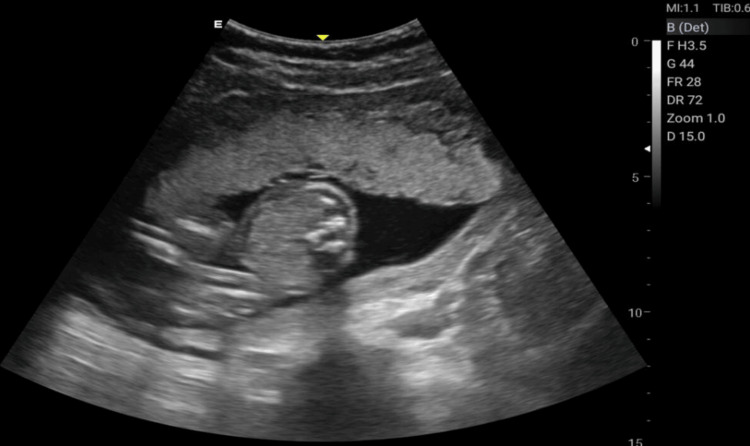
Placental ultrasound image.
